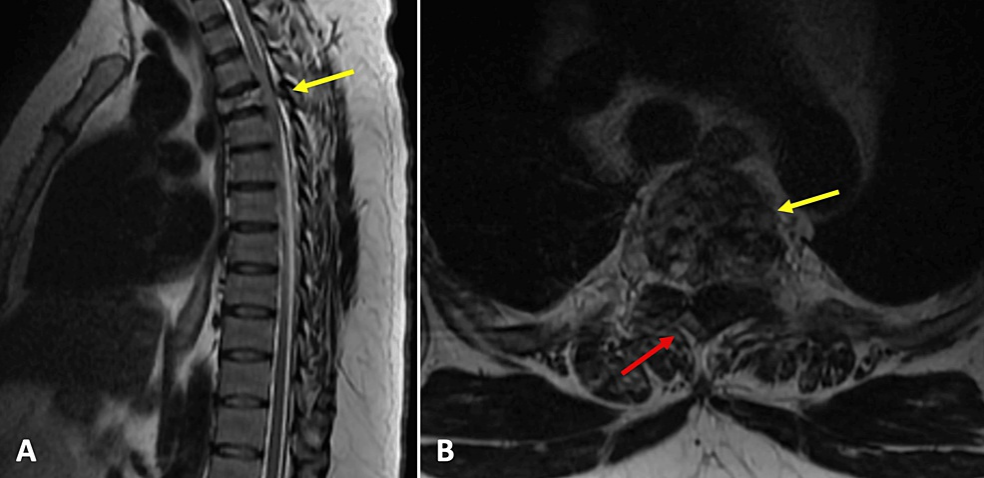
Pre-surgical magnetic resonance imaging (A) In the sagittal sections of T2-weighted MRI, a decrease in the signal of the T3 vertebral body lesion is observed, which is compressing the spinal cord (yellow arrow); (B) In the axial section of T2-weighted MRI, a lesion is observed that destroys the vertebral body (yellow arrow) and occupies the spinal canal, displacing neural tissue to the right (red arrow).
